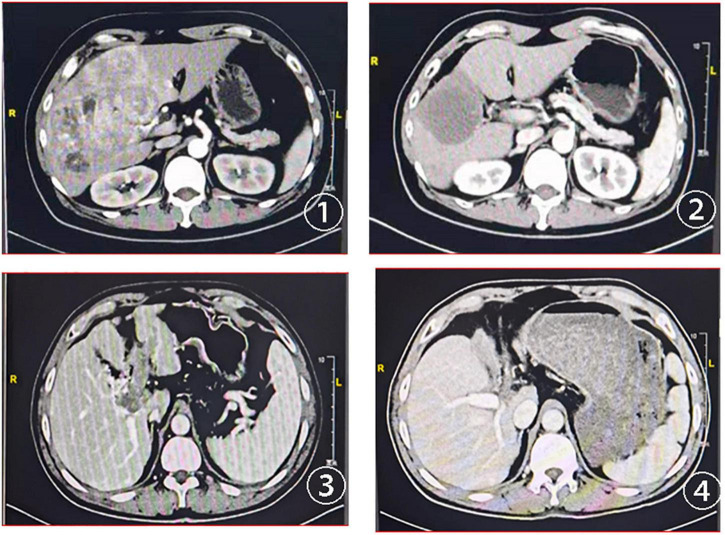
CT scans at baseline and after treatment assessment for two advanced patients with HCC treated with HAIC combined lenvatinib and PD-1. ① Baseline CT scans of the first patient; ② CT scans of the first patient after two cycles of the combined treatment; ③ baseline CT scans of the second patient with portal vein tumor thrombus; ④ the portal vein tumor thrombus disappeared after the combined treatment.
